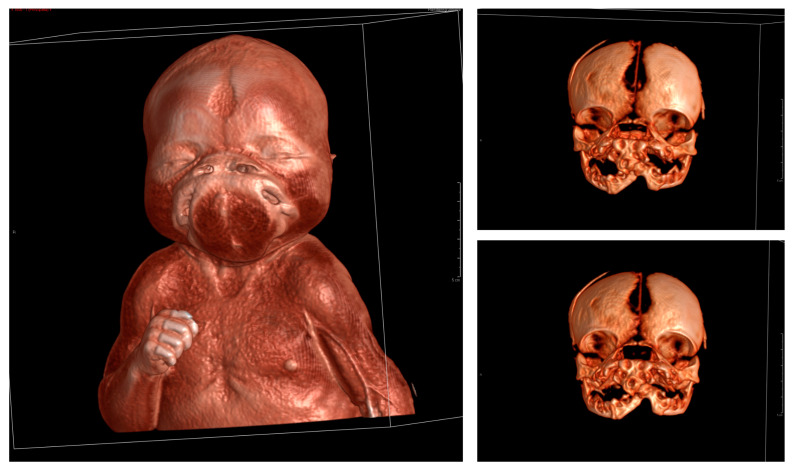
Cranial CT (3D volume rendering reconstruction): duplication of the mandible and, partially, of the maxilla, with partial duplication of the oral cavity and two distinct mouths. Note also the wide cranial sutures.
